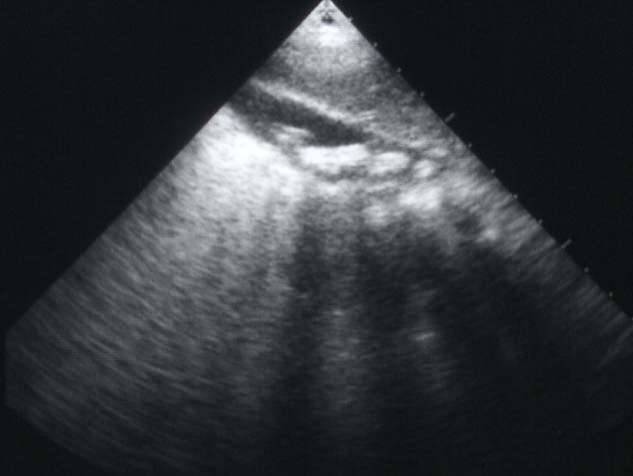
Abdominal ultrasound showing multiple gallstones.
